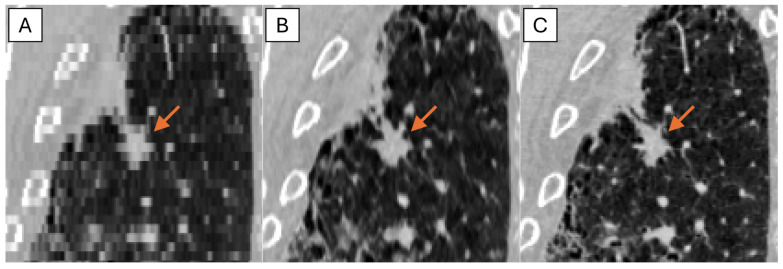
Coronal CT images of (A) thick-slice, (B) generated-thin-slice, and (C) thin-slice presenting GGNs as shown by the arrows.
